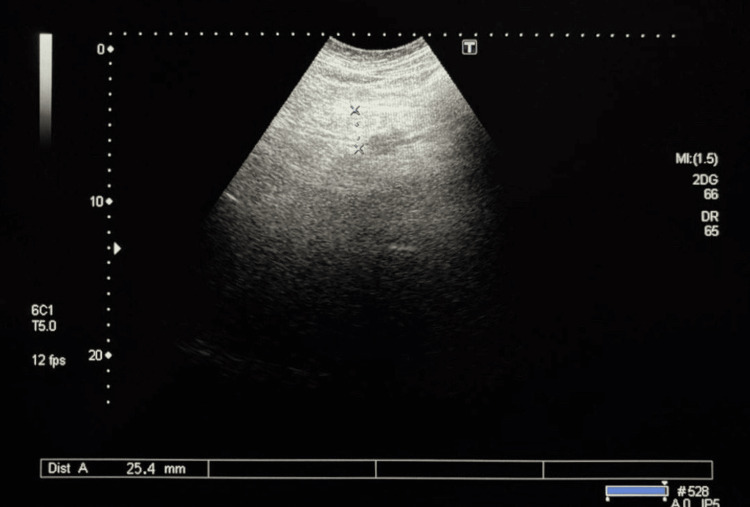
Ultrasound examination of the pancreas (the body of the pancreas) The body of the pancreas is visualized as a homogeneous structure with medium echogenicity and well-defined, smooth contours. The structure measures 25.4 mm in transverse section (blue dashed line; Dist A), which is consistent with the normal range for this anatomical region. The surrounding tissues exhibit normal echogenicity without signs of diffuse changes or focal lesions. No visible pathological inclusions, such as cystic or solid masses, are present.
